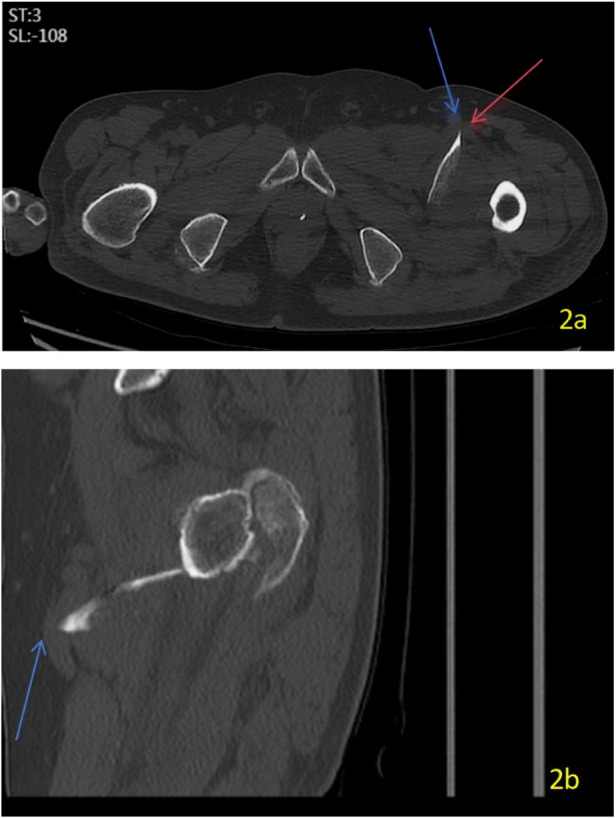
Two-panel CT series of the left hip. The left image (a, horizontal view) shows an anteriorly displaced bone spike from the lesser trochanter adjacent to vascular structures, indicated by a red arrow and a blue arrow. The right image (b, sagittal view) also shows this forward displaced spike adjacent to a vessel, marked by a blue arrow. This close relationship was the key imaging finding that prompted concern for potential vascular compromise.
